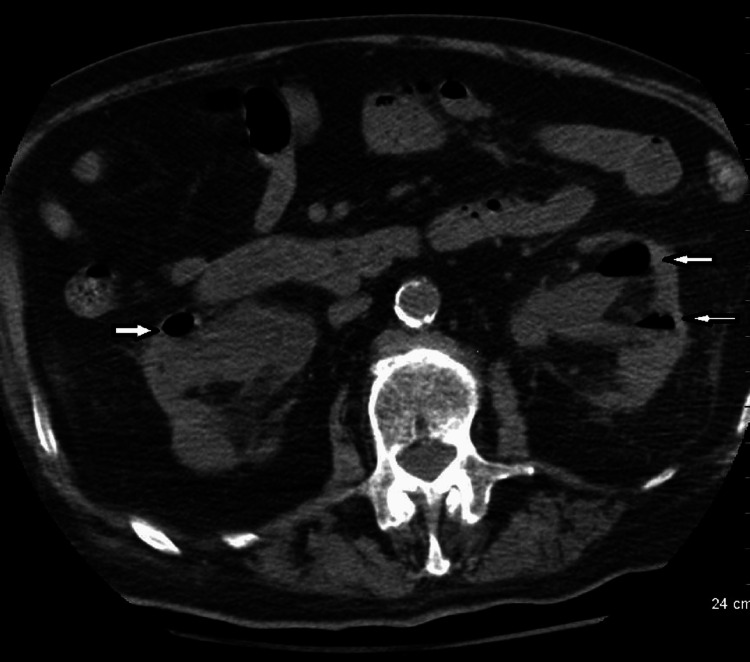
Non-enhanced CT image showing gas in both the right and left kidneys (white arrows).
